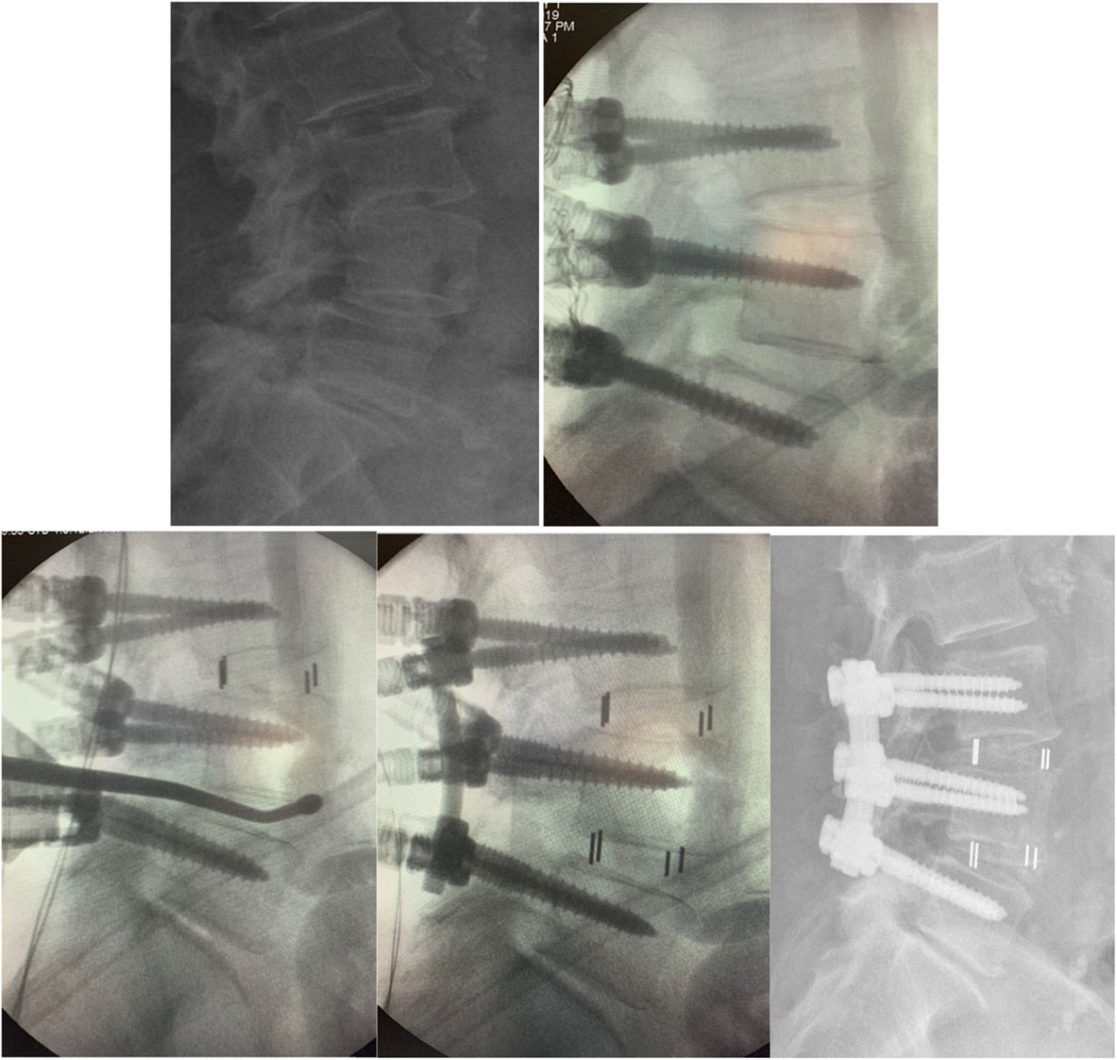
Female patient, 74 years old, diagnosed of lumbar degenerative spondylolisthesis and lumbar stenosis (L3/4 and L4/5). a, Forward slippage of L4; b, Pedicle screws were placed from L3 to L5; c, After decompression of both levels and cage insertion at L3/4, a lever repositioner was placed at the anterior rim of the slipped vertebrae under fluoroscopy; d, A rod was placed unilaterally and the pedicle screws of L3 and L5 were locked. After L4 was pried upward, the pedicle screw of L4 was locked and cage was then inserted; e, Postoperative X-ray showed satisfactory reduction of L4
